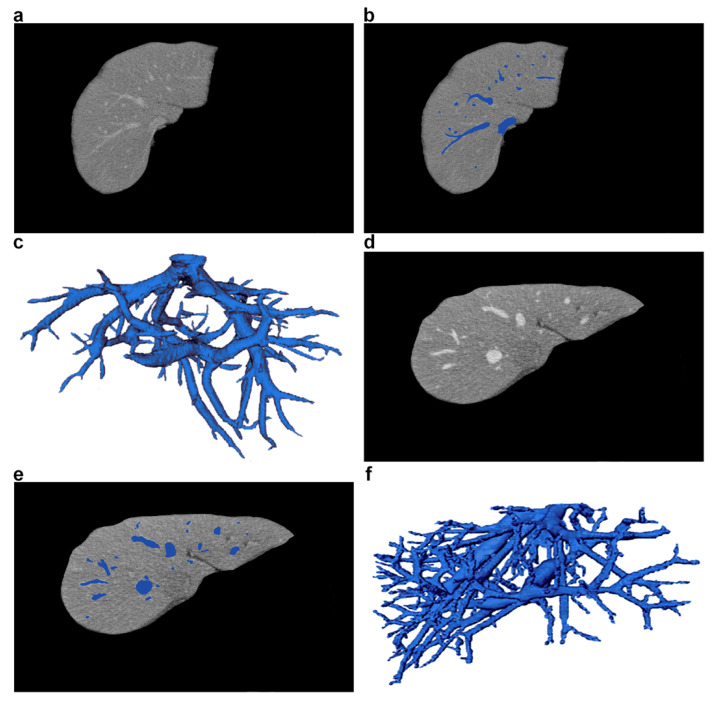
Sample CT images of liver and its blood vessels, taken from two different patients. (a,d) CT liver slices with vessels (axial view). (b,e) Vessel segmentation marked in blue. (c,f) 3D visualization of liver vessel trees.
